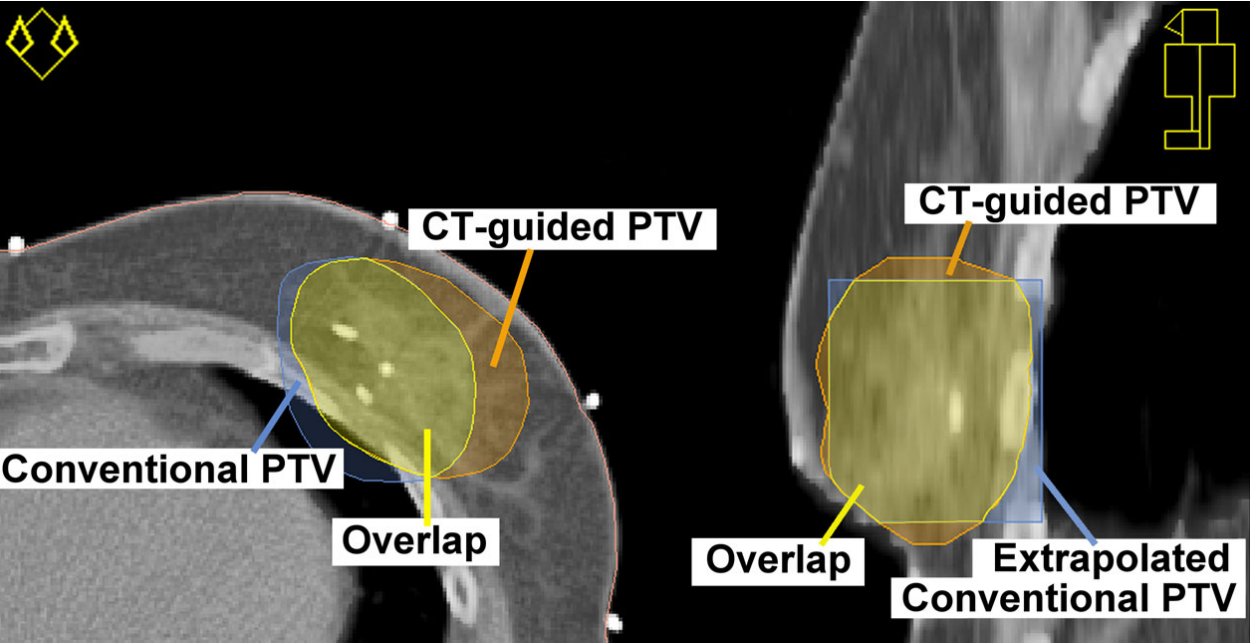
Conventional and CT-based boost planning target volume. Transversal (left) and sagittal (right) cross-sections of conventional and computed tomography (CT)-based boost planning target volume (PTV).
